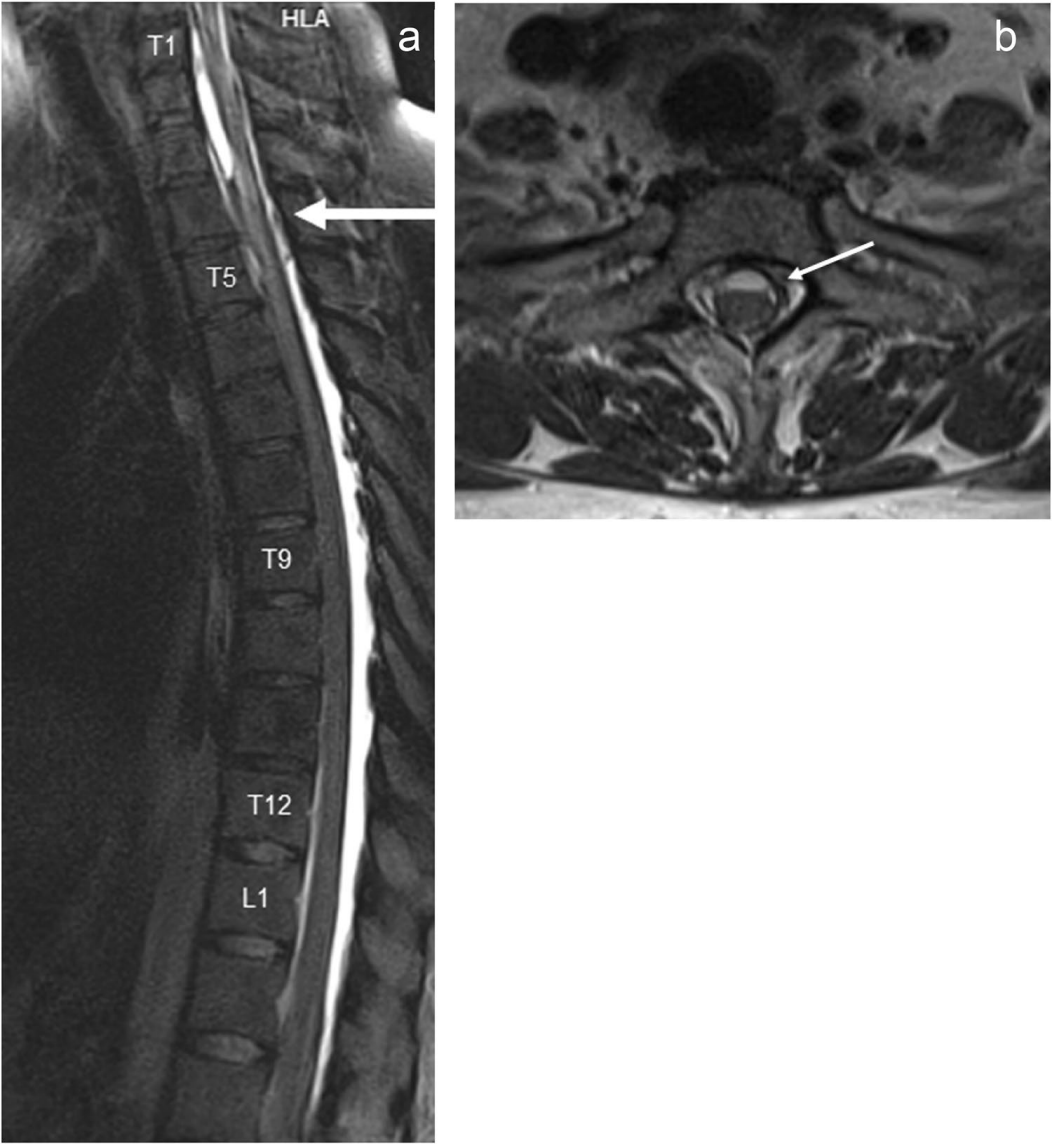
a MRI T2-weighted STIR-sagittal view of spine, b MRI T2-weighted STIR-transversal view of spine Arachnoid adhesions in spinal canal of the upper thoracic and lower cervical spine. Spinal sac and spinal cord deformities. Strict adhesion of the spinal cord to the front wall of dura mater at the Th2–Th3 level with a coexisting malacia
